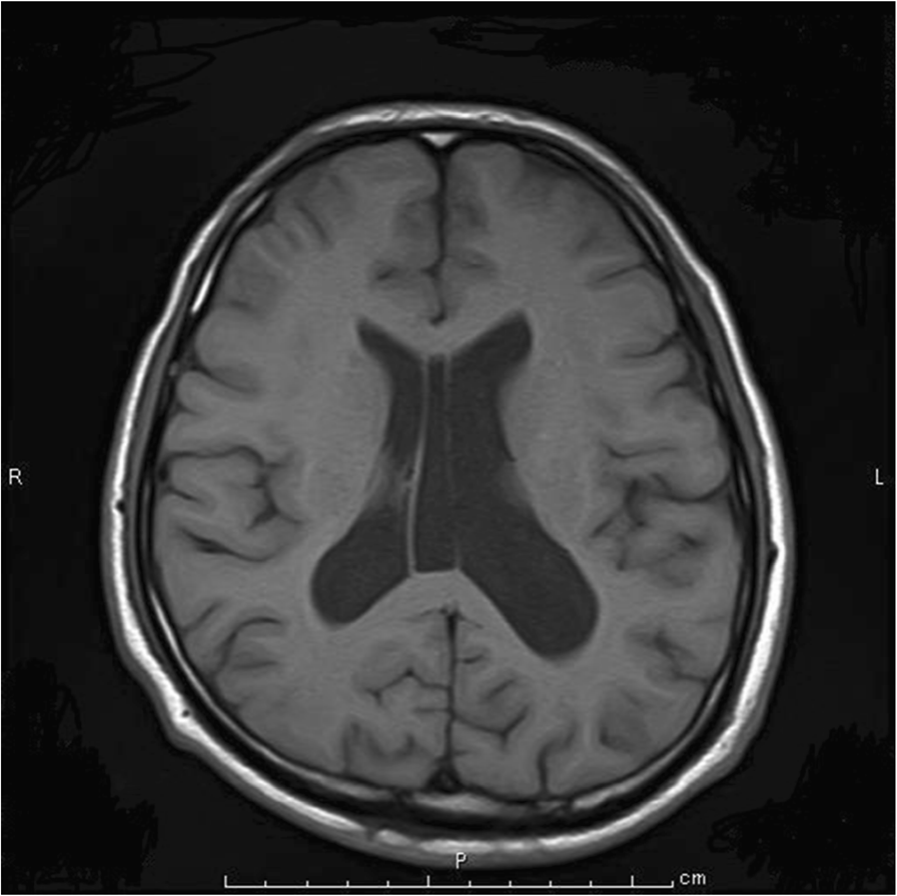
MRI of the brain and dilated lateral ventricle.
